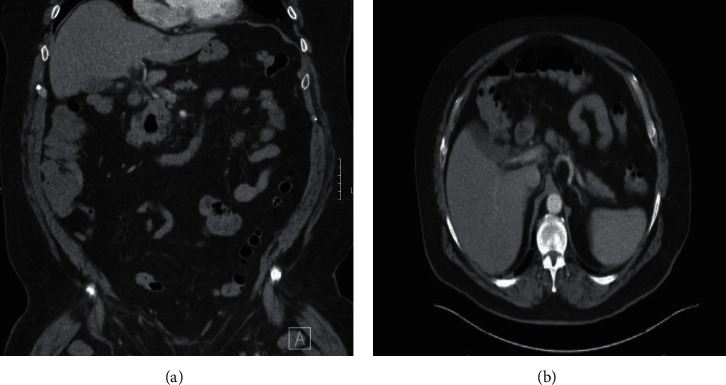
(a), (b) CT scan at initial presentation: moderate stool burden, no other findings.
